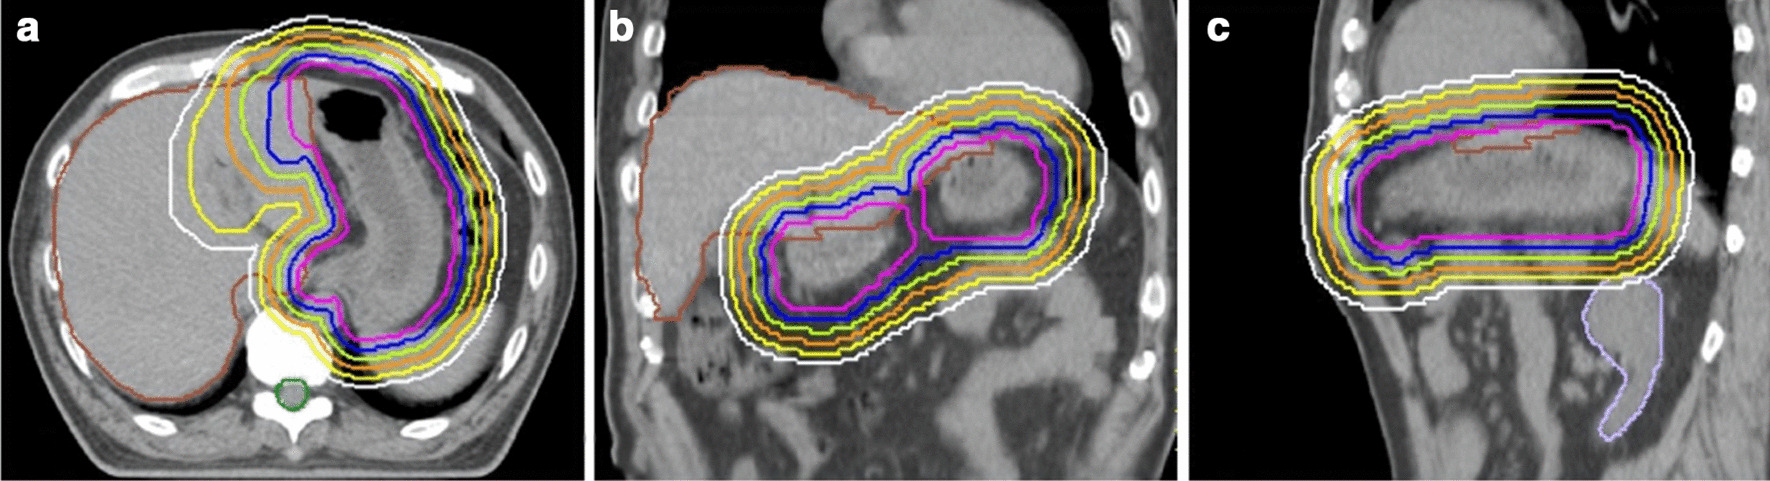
PTV structures for the retrospective evaluation. PTV structures with 5 mm (purple), 10 mm (blue), 15 mm (yellow-green), 20 mm (orange), 25 mm (yellow), and 30 mm (white) margins from the CTV delineated based on the 4D-CT images (CTV-4D) are displayed on the axial (a), coronal (b), and sagittal (c) planes of planning CT images (AIP CT images of 4D-CT). The OARs of the liver, spinal cord, and kidneys are shown in brown, green and lavender, respectively
